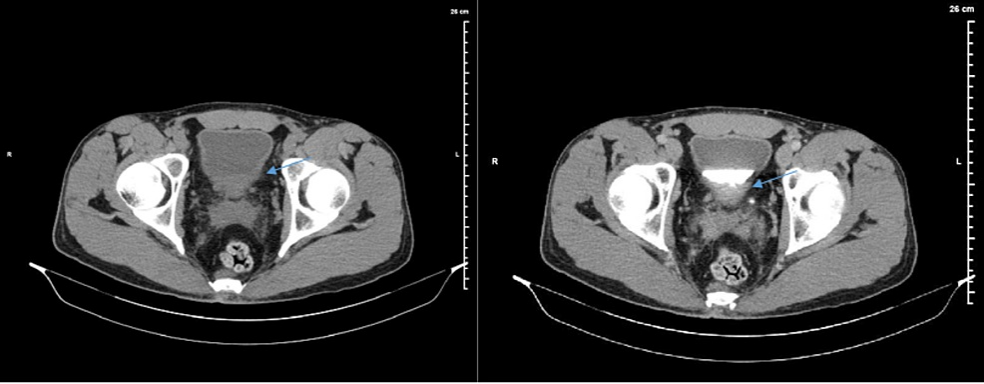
Left: Noncontrast CT transverse image of the bladder. Blue arrow is pointing at the thickened posterior bladder wall. Right: CT urogram, nine minutes delayed transverse image of the bladder. Blue arrow is showing posterior wall enhancement. CT: computerized tomography
